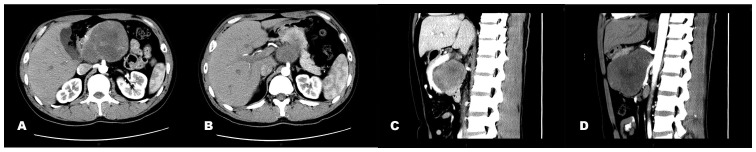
CT scan presents a 10-cm heterogeneous retroperitoneal solid mass on the (A) abdominal aorta and (B) closely attached to the posterior wall of the pancreas. The mass is surrounded by the (C) portal vein, (D) celiac trunk and superior mesenteric artery.
